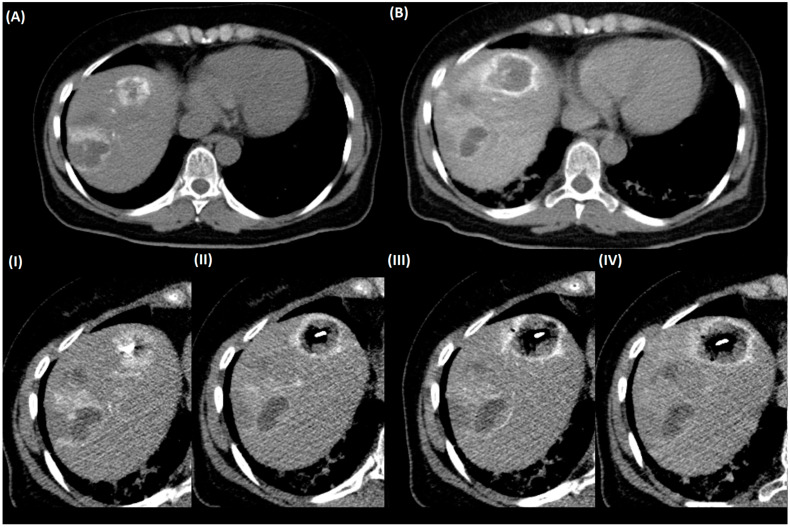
CT scan in a patient with contrast medium administration via an IA catheter in the common hepatic artery during ablation of a colorectal liver metastasis. (A) Planning scan before ablation, (B) final scan after ablation, (I–IV) control images during ablation with administration of 2–4 mL of contrast medium IA show growing of the ablation area.
